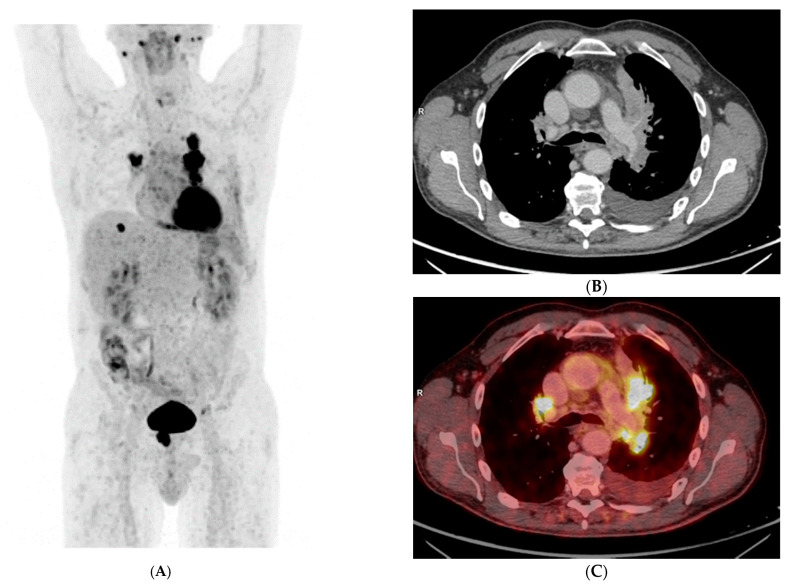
(A) Maximum Intensity Projection (MIP) FDG-PET showing multiple FDG-avid lesions, (B) transaxial CT image showing a tumor atelectasis complex in the left upper lung’s lobe and enlarged lymph nodes in both hila, (C) same as (B) fused with FDG-PET.
